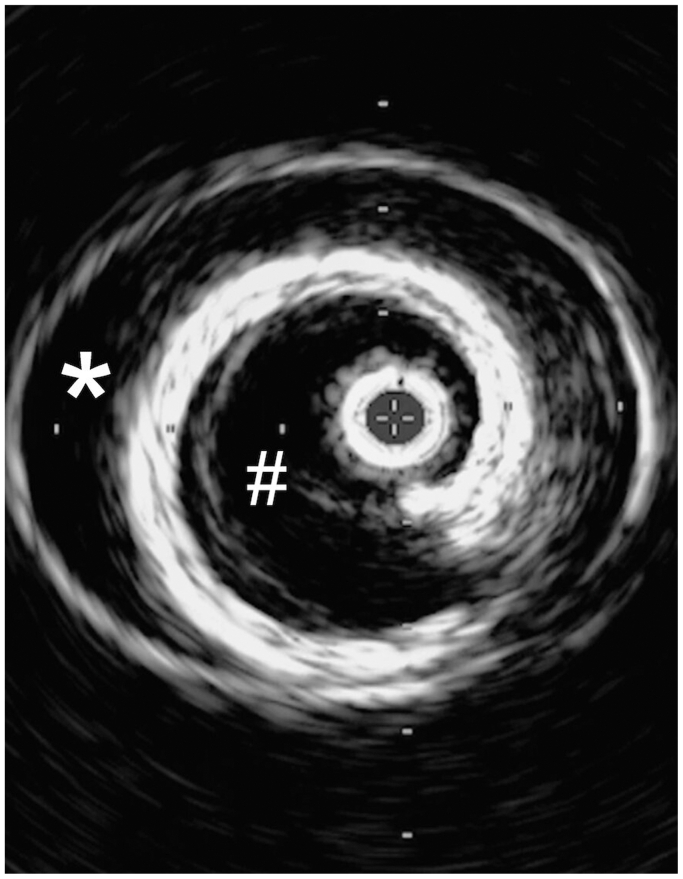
Intravascular ultrasound image portraying the creation of two lumens within the aorta. The *star* shows the false lumen of the aortic wall, whereas the *pound sign* marks the true lumen created by the Dacron graft (DG) within the aorta.
